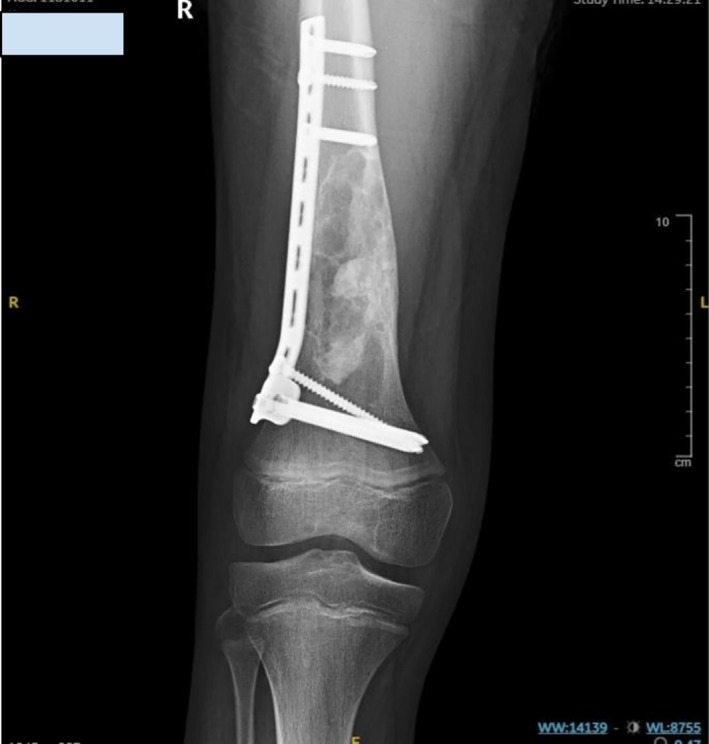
Four‐month follow‐up anteroposterior radiograph showing interval healing and graft incorporation after ORIF. There is progressive osseous remodeling with incorporation of graft material within the prior curettage defect. Alignment is maintained, fixation hardware remains intact, and there are no radiographic signs of implant loosening or recurrent lesion.
